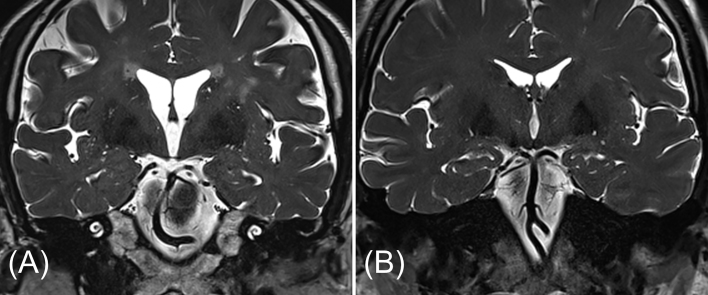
Assessment of the tortuosity of the basilar artery on coronal T2-weighted images. A, Tortuous basilar artery with marked displacement to the right. B, Straight basilar artery in the midline.
